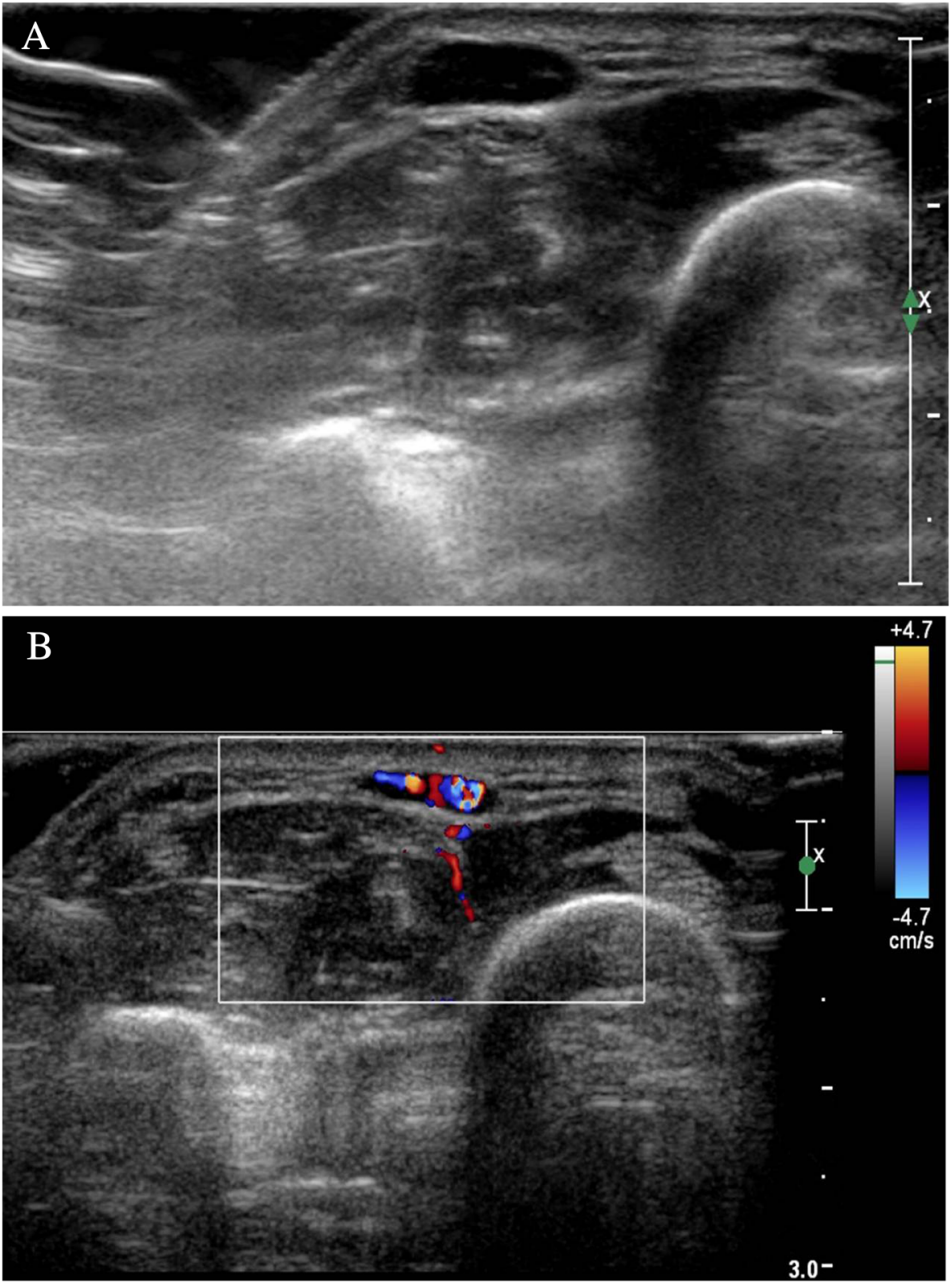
Ultrasonograms of the mass lesion. a Ultrasonogram of the forearm shows a well-defined, hypoechoic, 0.9cm soft tissue nodule. b Soft tissue mass with increased vascularity
